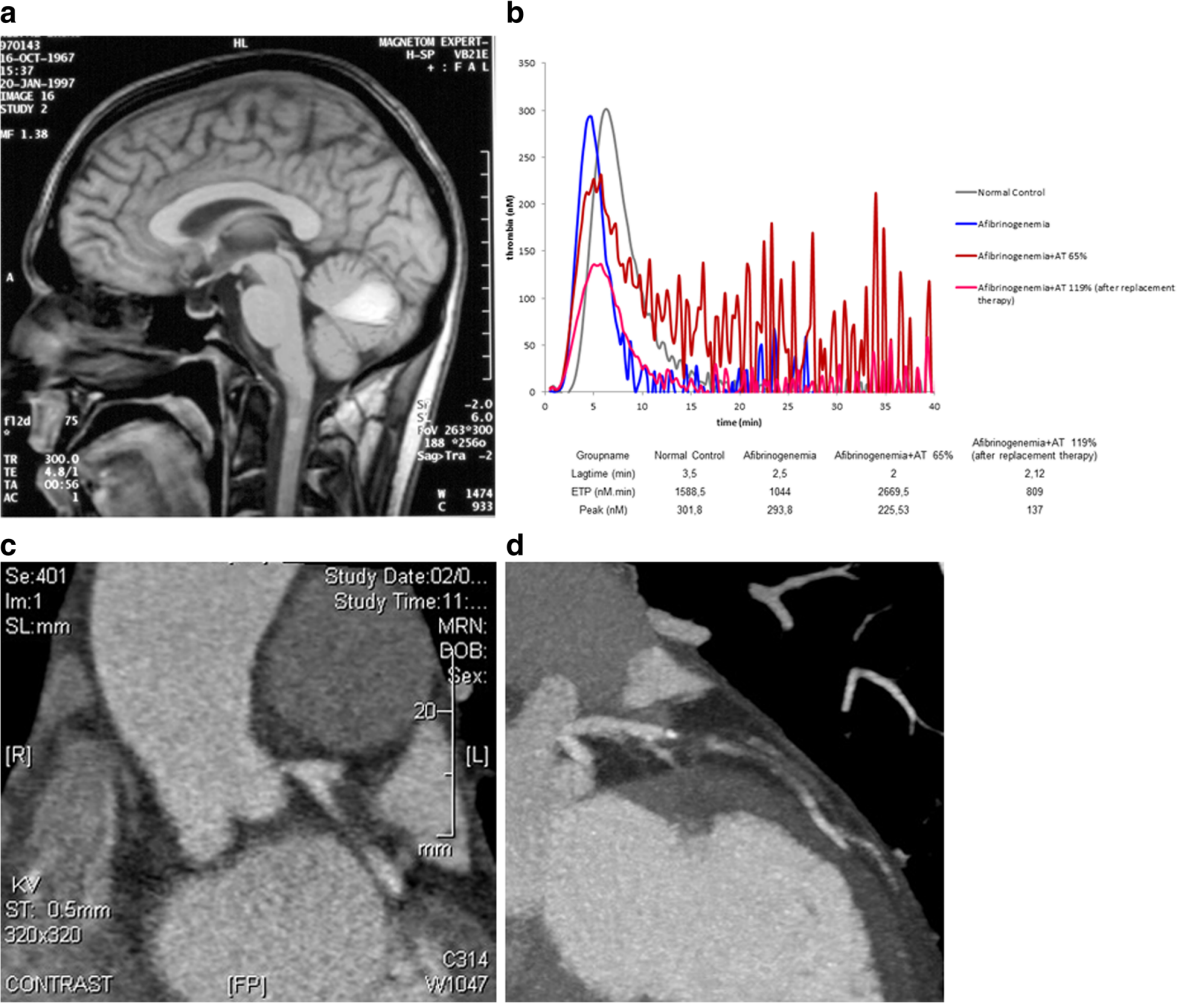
a Sagittal computed tomography angiography showing a left cerebellar hemisphere hemorrhage. b Thrombin generation curves obtained with 5pM tissue factor and 4 μM phospholipids (final concentration) in platelet-poor plasma using calibrated automated thrombin generation assay (Stago, Asnières, France). The area under the thrombin generation curve (or endogenous thrombin potential) is significantly higher in the patient (red) compared to another subject with afibrinogenemia (blue) or a representative normal control (grey). In this patient with combined inherited antithrombin and fibrinogen deficiency, increased thrombin generation is due to insufficient inhibition of thrombin. Thrombin generation is decreased after infusion of 30 U/kg antithrombin concentrate (pink). c Visualization of the left coronary artery with computed tomography coronary angiogram showing 80% stenosis in the common trunk. d Visualization of the left coronary artery with computed tomography coronary angiogram showing 50% stenosis in the anterior interventricular branch of left coronary artery
